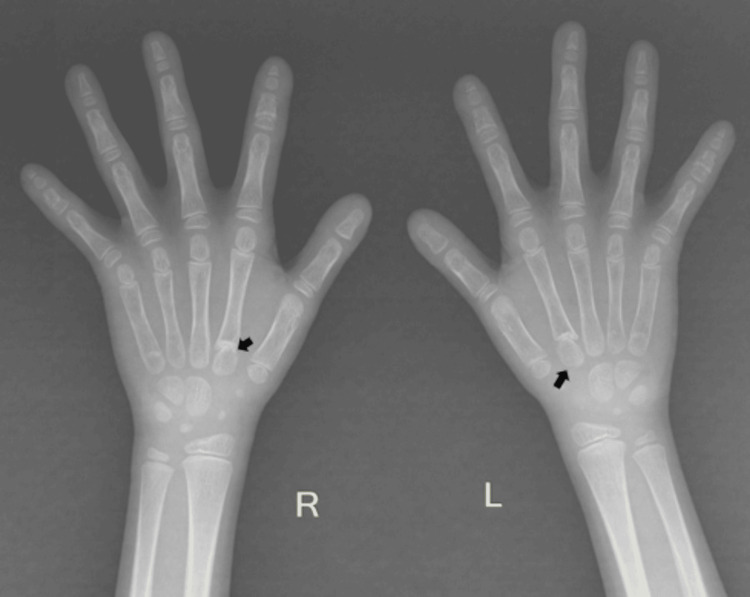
Radiograph of both hands of this seven-year-old girl showing short and pointed distal phalanges. Pseudoepiphyses (black arrows) are noted at the base of the metacarpals of both index fingers.
